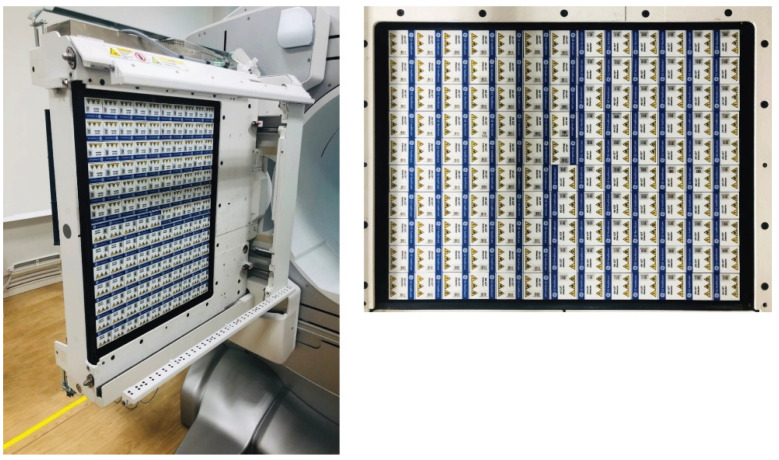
GE Discovery: CZT SPECT device. Figure from [11]. Representation of the detector arrangement for a general-purpose CZT device.
